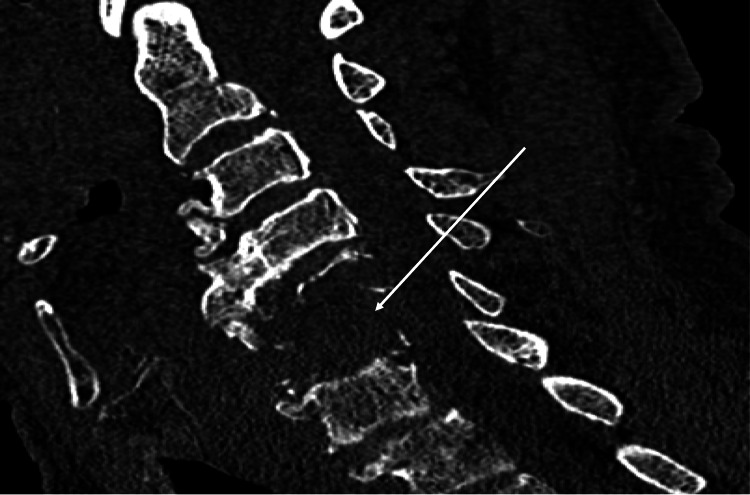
C5/C6 lesion (white arrow) CT scan depicting C5/C6 cervical bones with aggressive soft tissue mass causing complete destruction of the fifth and sixth cervical vertebra
